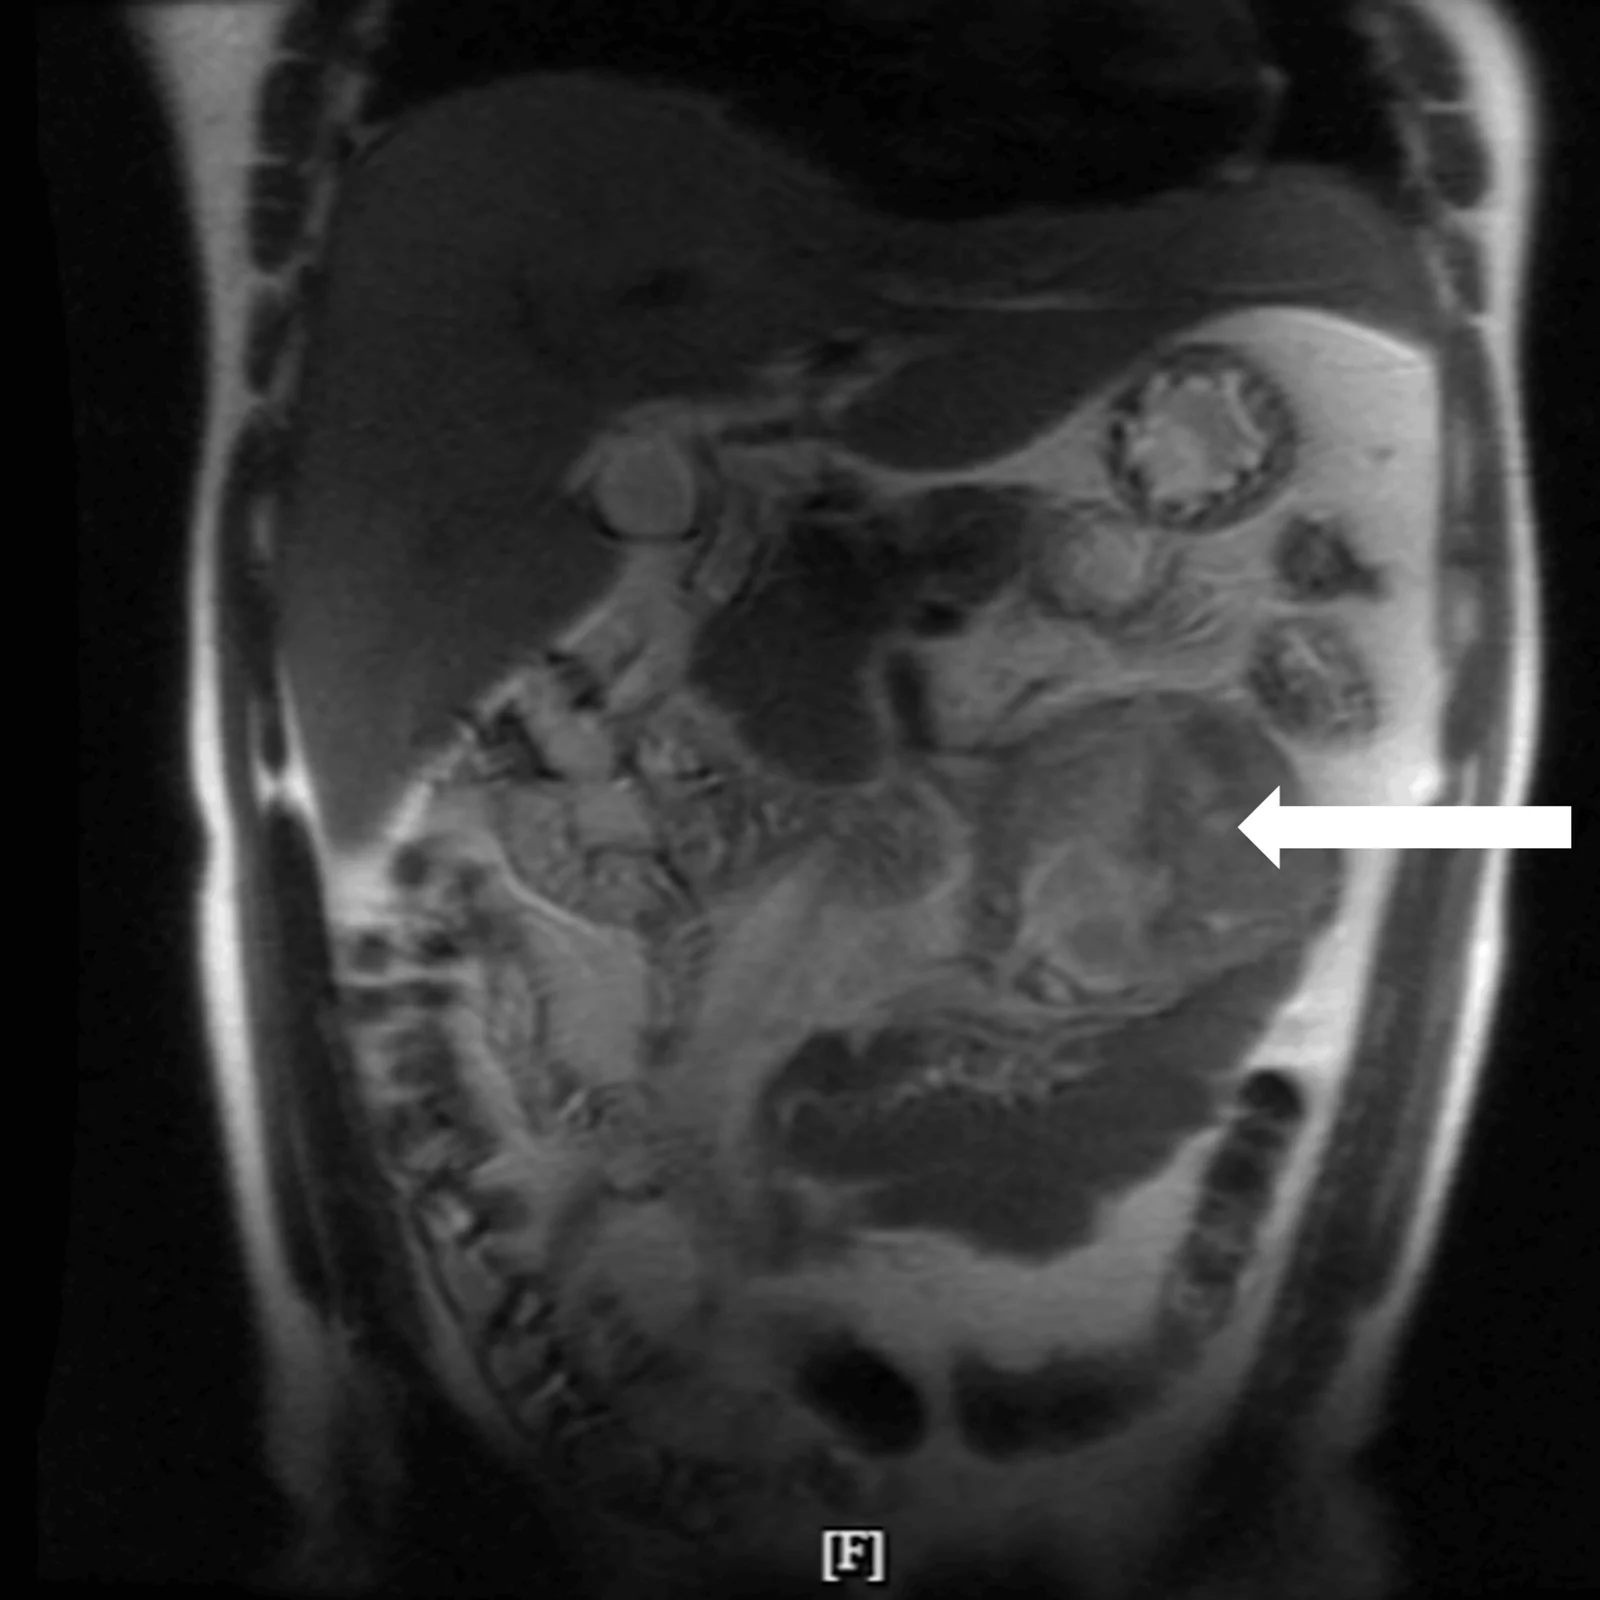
Coronal MRI image demonstrating a heterogeneously enhancing mixed cystic-solid lesion (white arrow) within the mesentery, abutting the small bowel
